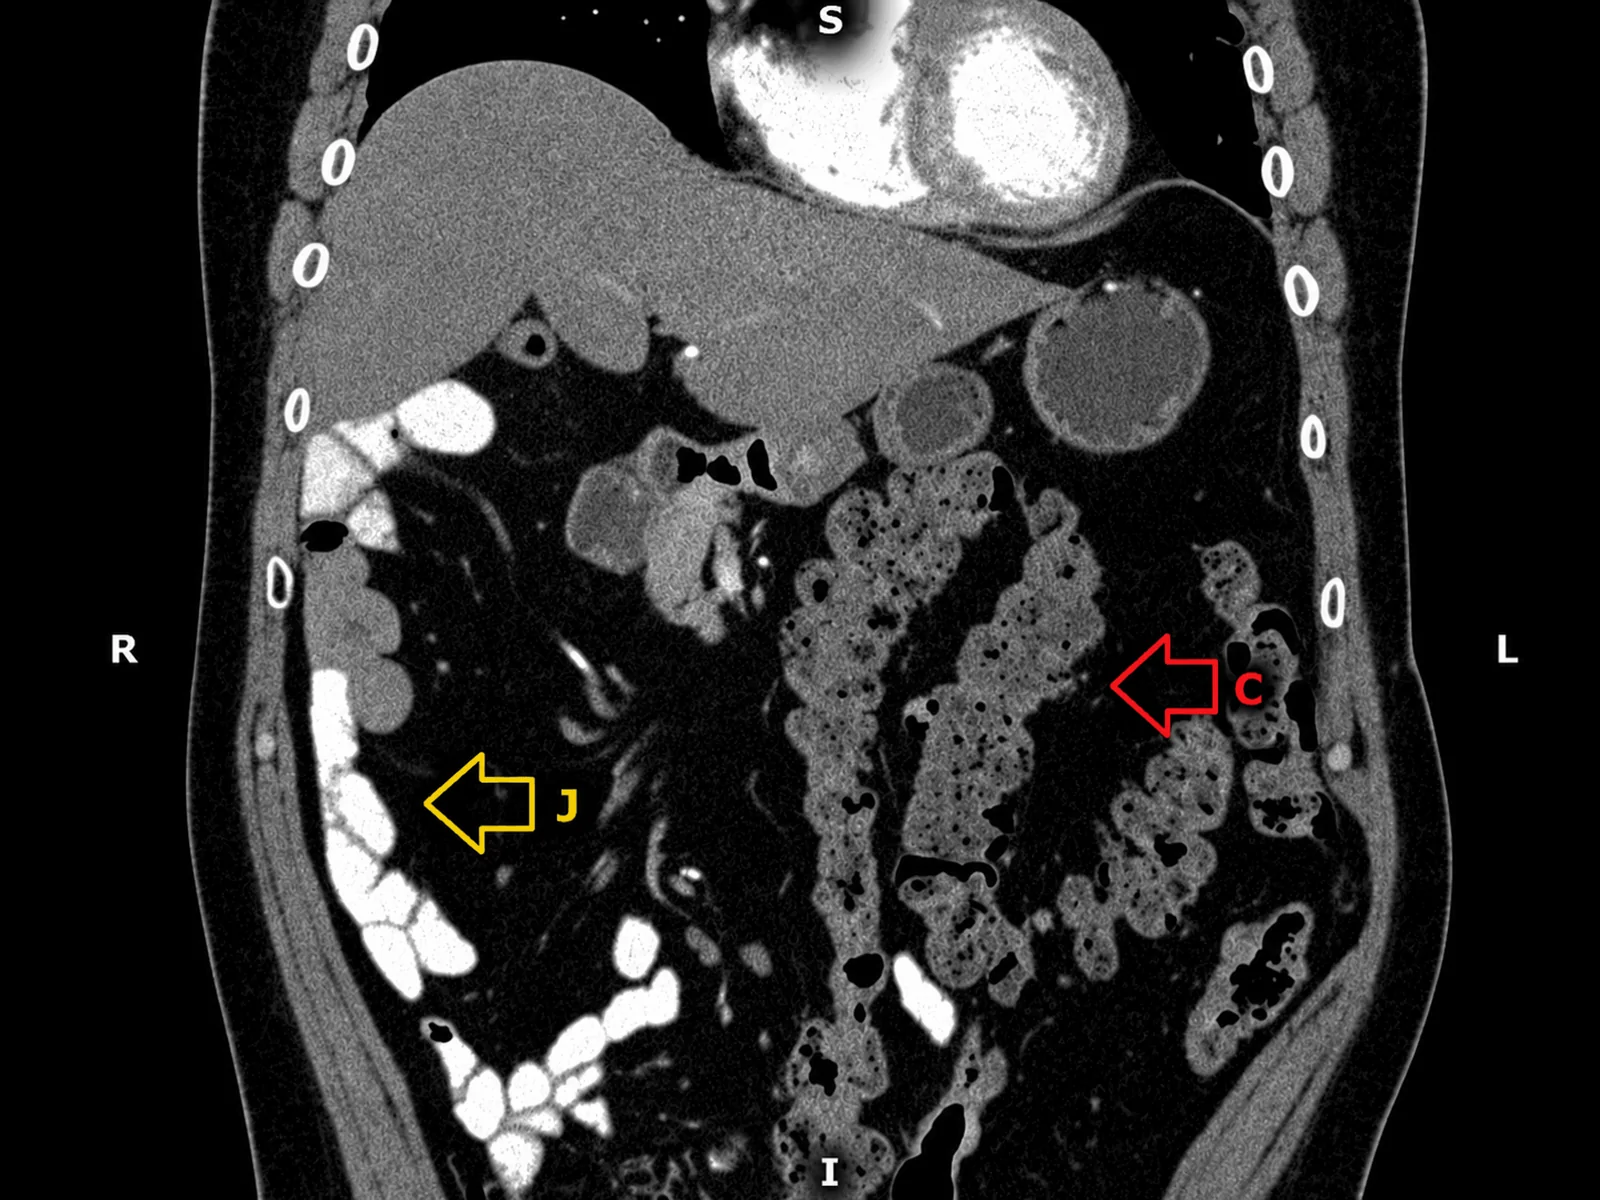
Coronal contrast-enhanced CT image of patient 1 demonstrating right-sided predominance of the duodenum and jejunal loops, a midline course of the ascending colon, and a predominantly left-sided location of the remaining colon. The yellow arrow labeled J indicates the jejunal loops, and the red arrow labeled C indicates the colon. R and L indicate the patient’s right and left sides, respectively, while S and I indicate the superior and inferior directions. CT: computed tomography
